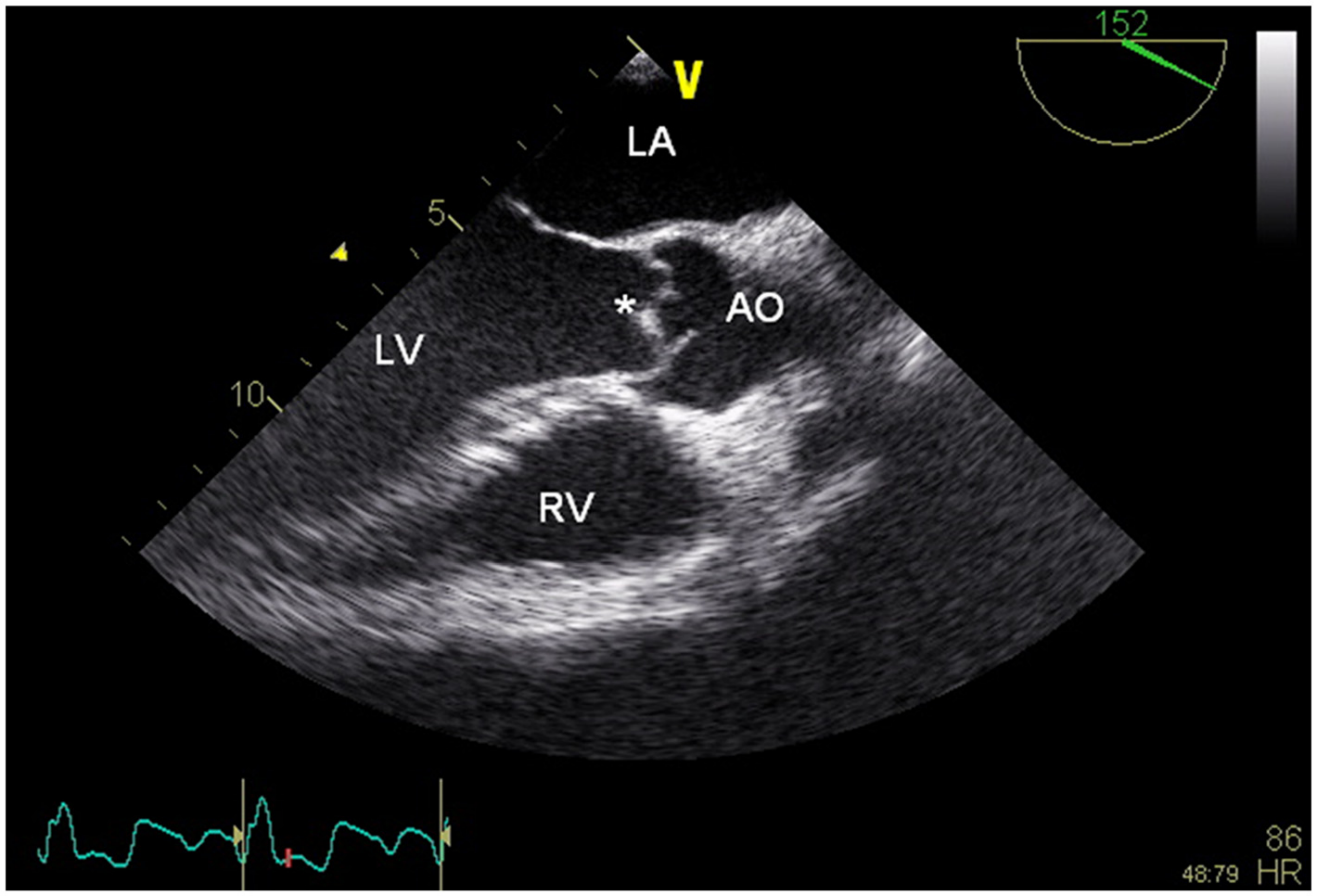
Transesophageal echocardiography shows a vegetation on the noncoronary cups of the aortic valve. LA: left atrium, LV: left ventricle, RV: right ventricle, AO: aorta, *:vegetation.
